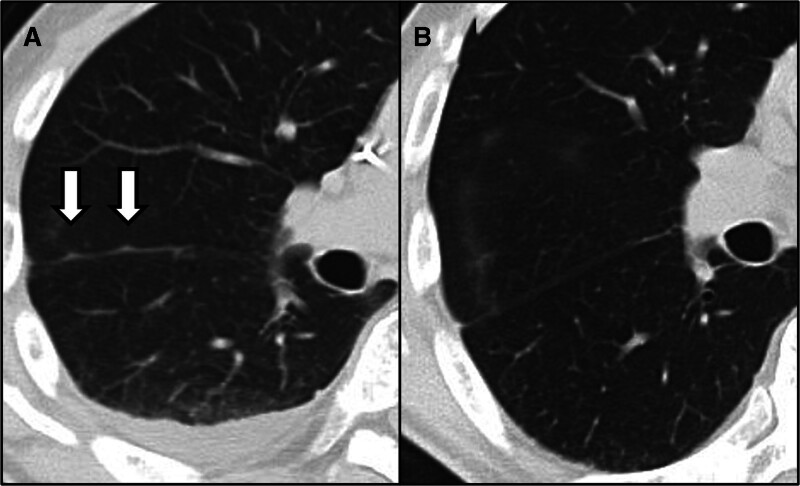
Radiologic findings of pleural dissemination and response to chemotherapy. (A) Chest CT performed at recurrence revealed multiple small nodular opacities along the interlobar pleura, raising suspicion of pleural dissemination. (B) CT imaging obtained 5 months after chemotherapy re-initiation showed complete radiological disappearance of the nodular lesions.
